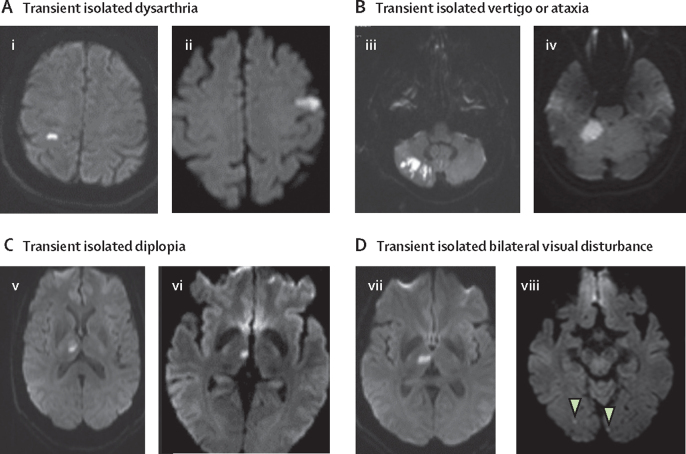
MRIs of sudden onset monosymptomatic non-consensus TIAs in eight different patients with a causative ischaemic lesion (A) Two patients with transient isolated dysarthria. (i) 59-year-old patient who had 30 s episode of slurred speech; examination normal; MRI shows restricted diffusion in right post-central gyrus; MRA normal; grade 2 shunt on bubble TCD. (ii) 64-year-old patient who had sudden onset isolated slurred speech for 12 h, which fully resolved; examination normal next day; MRI shows restricted diffusion in left parietal cortex; MRA normal; grade 2 shunt on bubble TCD. (B) Two patients with transient isolated vertigo or ataxia. (iii) 65-year-old patient with sudden onset isolated vertigo, which lasted 3 min; several recurrences over next few hours; examination showed nystagmus on left lateral gaze; CT brain scan in emergency department was normal; all symptoms resolved after 6·5 h; no hearing loss or tinnitus, but reported episodes of isolated unsteadiness lasting a few seconds, occurring daily for several weeks; MRI shows partial right PICA territory infarct, with tight stenosis of the proximal right vertebral artery on MRA. (iv) 45-year-old patient, heavy smoker, with sudden onset of rotatory vertigo and nausea lasting 12 h; examination normal the following day; MRI shows restricted diffusion in right cerebellum; MRA was normal. (C) Two patients with transient isolated double vision. (v) 64-year-old patient with sudden onset of double vision lasting 30 min; could see normally if covered either eye; saw an optician the following day and no abnormalities were detected; MRI shows restricted diffusion in right thalamus; MRA normal; grade 2 shunt on bubble TCD. (vi) 58-year-old patient woke up with diplopia, with one image diagonally above the other, which resolved on closing either eye; lasted 90 min but had mild headache for 24 h; past history of anxiety and migraine; examination normal; MRI shows restricted diffusion in right thalamus. (D) Two patients with transient isolated bilateral visual disturbance. (vii) 61-year-old patient with sudden onset blurring of the whole visual field; vision was not double; symptoms fully resolved after 30 min; examination normal; MRI shows restricted diffusion in right thalamus; MRA normal; grade 2 shunt on bubble TCD. (viii) 74-year-old with sudden onset blurring of whole visual field lasting 15 min; examination normal; MRI shows bilateral restricted diffusion in occipital lobes (arrows); CT angiography showed bilateral vertebral artery stenosis. MRA=magnetic resonance angiography. TCD=transcranial doppler. PICA=posterior inferior cerebellar artery.
